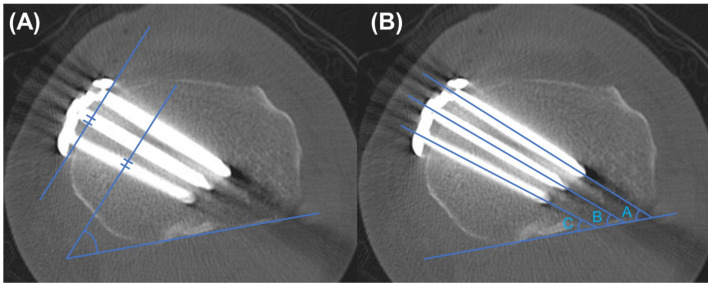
Measurement of the plate angle and screw angles on the CT axial scan. (A) The plate angle was defined as the angle between the anteroposterior line connecting the proximal part of the plate and the posterior tibial condylar line. (B) The A, B, and C screw angle was defined as the angle between the line drawn along the screw and the posterior tibial condylar line. (A, B, and C screws from anterior to posterior, respectively).
